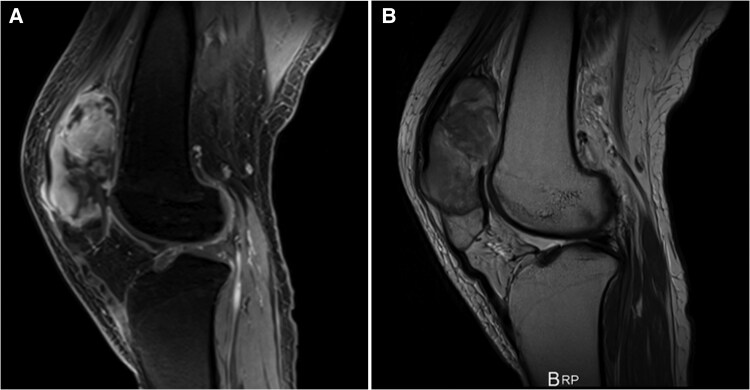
Preoperative contrast-enhanced magnetic resonance (MR) imaging of the right patella revealed a lobulated mass. *A*, Sagittal T1-weighted MR image. *B*, Sagittal T2-weighted MR image.
